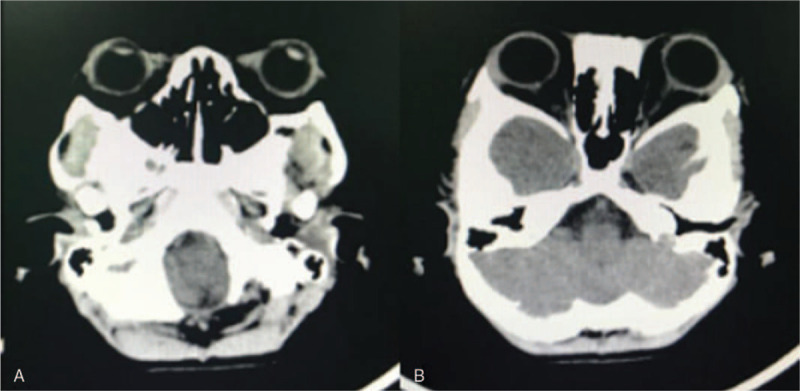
Postoperative computed tomography scans 1 year after hematoma removal and left blepharorrhaphy. (A) and (B) No hematoma recurrence.
